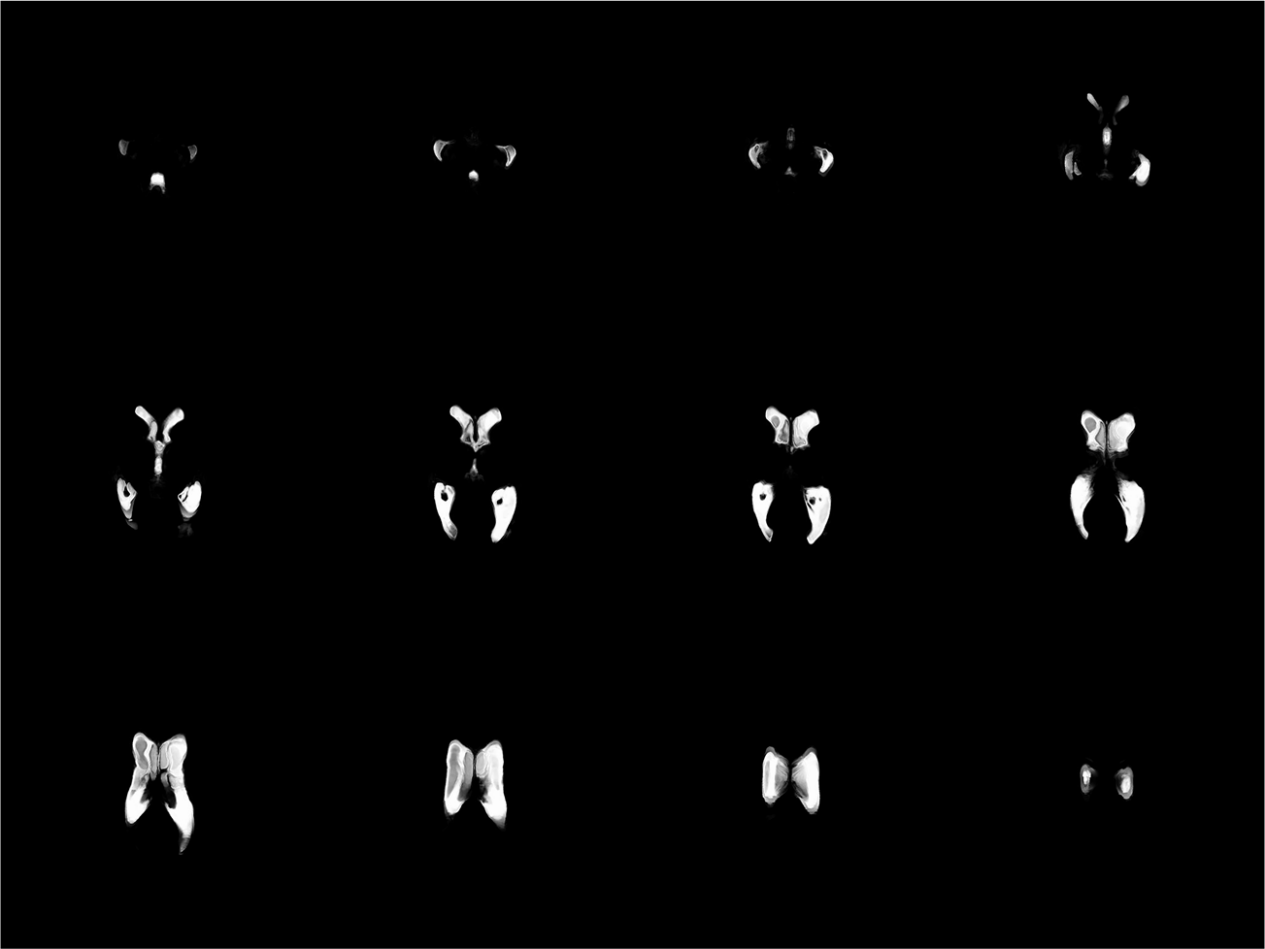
MRI segmentation in a boy born at 28 weeks of gestation who developed post-haemorrhagic ventricular dilatation and was examined at a postnatal age of 12 weeks. The montage of images shows the MRI segmentation of the ventricles in several transaxial slices
